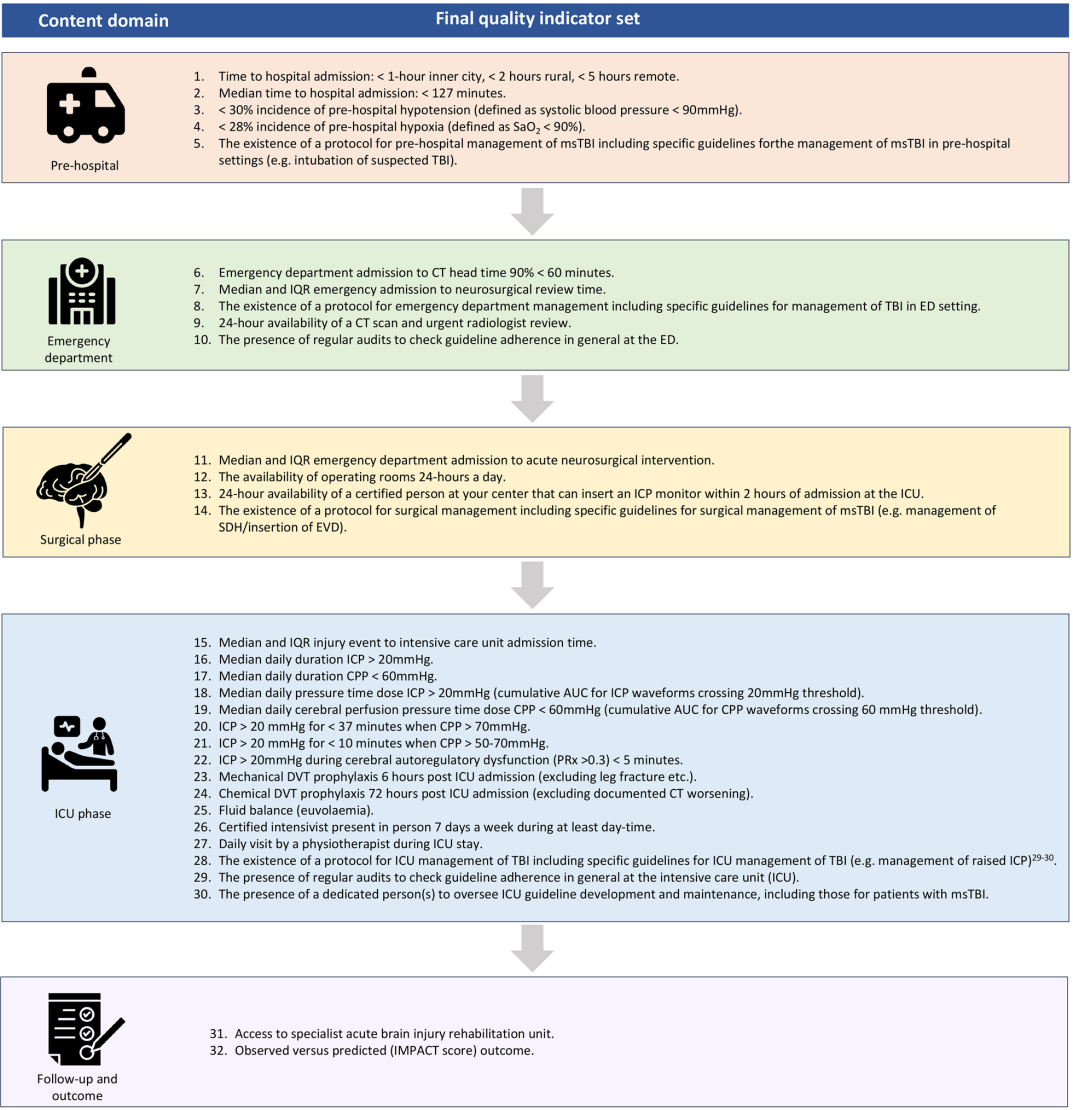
List of final quality indicators set for acute management of msTBI in Australia. AUC, area under the curve, CPP, cerebral perfusion pressure, CT, computed tomography, DVT, deep vein thrombosis, EVD, extra ventricular drain, ICU, intensive care unit, ICP, intracranial pressure, IQD, interquartile range, msTBI, moderate to severe traumatic brain injury, PRx, pressure reactivity index, SDH, acute subdural hematoma, TBI, traumatic brain injury
